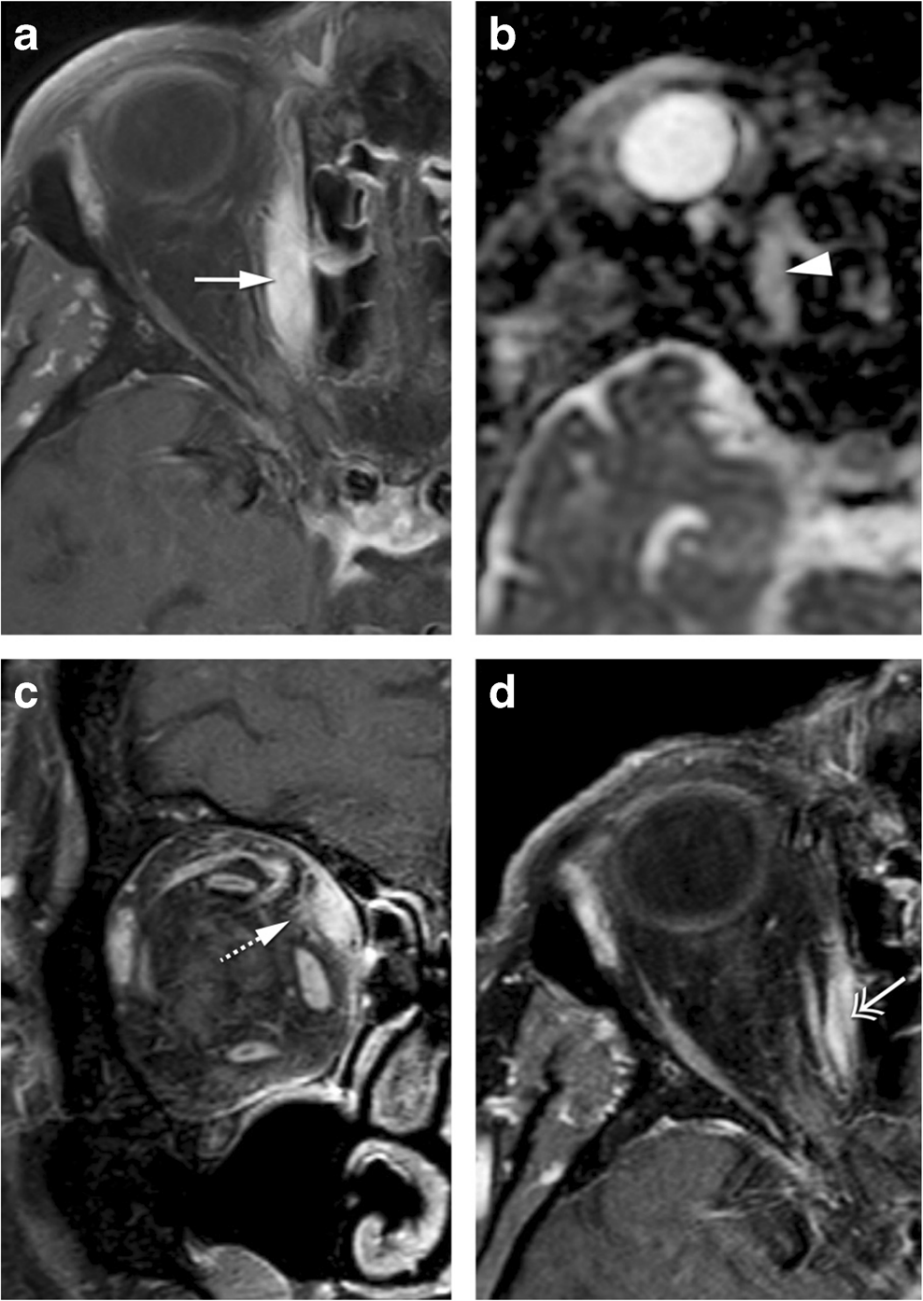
68-year-old male. Inflammatory myositis of the right superior oblique muscle. Enhanced axial T1-WI with fat signal suppression (a, d), axial ADC (b), and enhanced coronal T1-WI with fat signal suppression (c): enlarged and with marked contrast enhancement right superior oblique muscle (arrow), with no DWI restriction (arrowhead), and slight surrounding cellulitis (dashed arrow), favoring inflammation. Clinically not suspicious for infection. Improvement after corticosteroids (d) avoiding biopsy (double-headed arrow)
